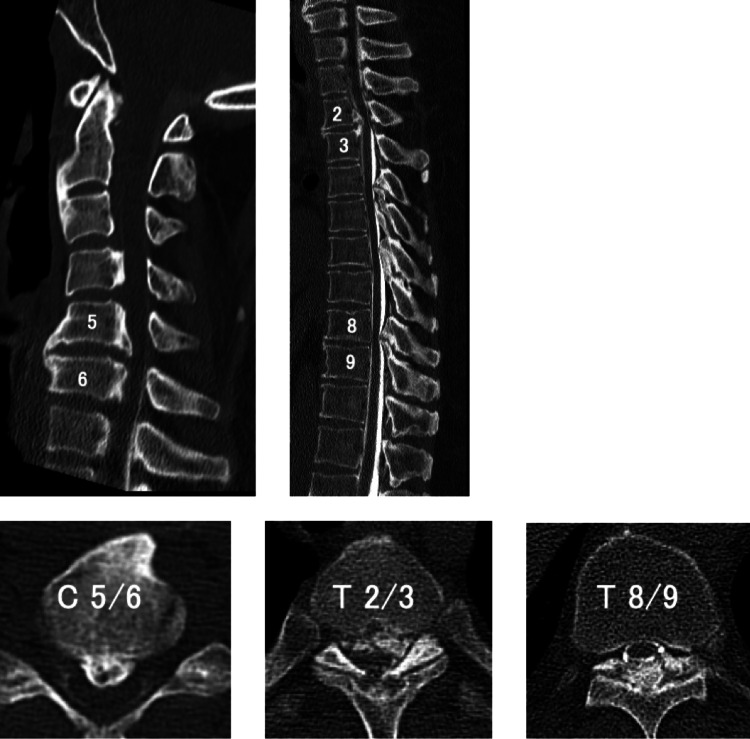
Computed tomography myelography of the spine showed ossification of the posterior longitudinal ligament from C3 to C7 and at T2/3. Ossification of the ligamentum flavum was noted from T4 to T10.
